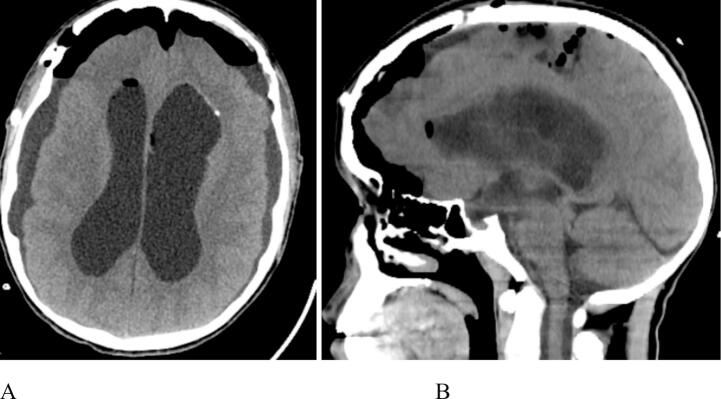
Postoperative axial [A] and sagittal [B] CT scans without contrast show near-total tumor removal with a small remnant attached to the ventricular wall. The scans also reveal a marked reduction in ventricular size, bilateral frontal pneumocephalus, and bilateral subdural collections, which were managed with a temporary subdural drain.
